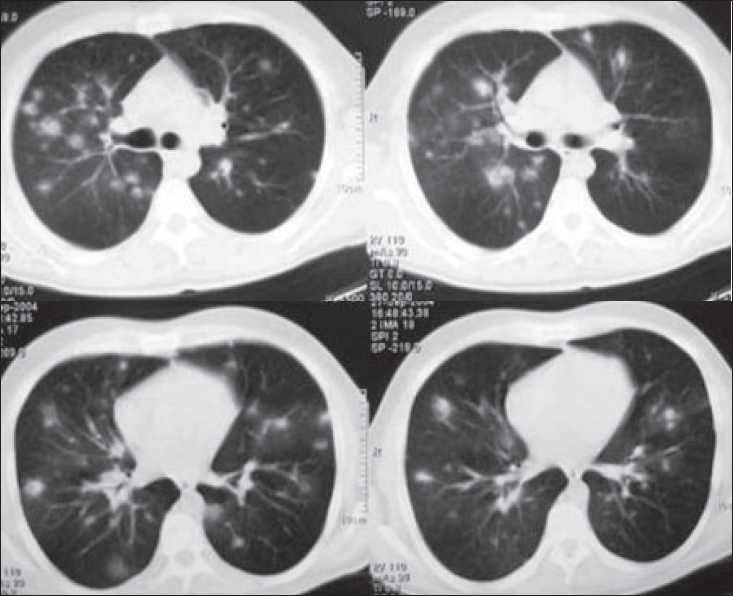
Thorax CT demonstrating multiple patchy and nodular infiltrations
